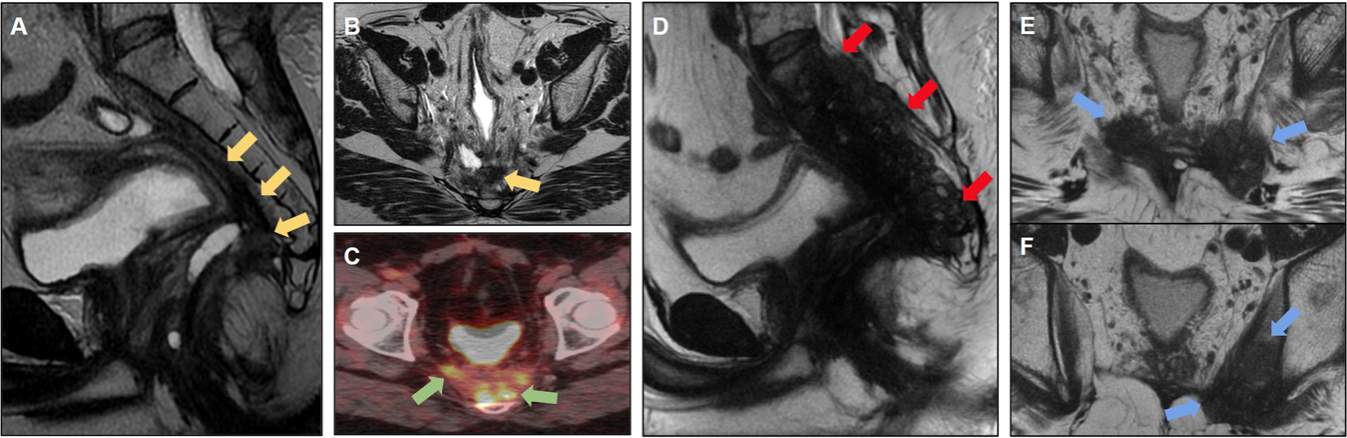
A Sagittal and (B) axial T2-weighted images; the patient underwent abdominoperineal amputation for low rectal cancer with positive circumferential resection margin, developing poorly defined tissue in the posterior pelvic space (yellow arrows), suspicious for neoplastic recurrence. C Positron emission tomography (PET)-computed tomography (CT) demonstrating increased glycolytic metabolism (fluorodeoxyglucose (FDG)) in the presacral tissue and internal iliac arteries, consistent with neoplastic disease (green arrows). No distant metastasis was observed; however, surgery was contraindicated due to the patient’s clinical condition. D Follow-up evidence of an unresectable lesion infiltrating the sacrum and invading the bilateral superior sacral foramen, left lumbosacral plexus, and spinal canal. The lesion extends into the presacral space, causing retraction of the bladder and internal iliac vessels to the left (red arrows). E and F also show extension into the lateral pelvic spaces (blue arrows)
